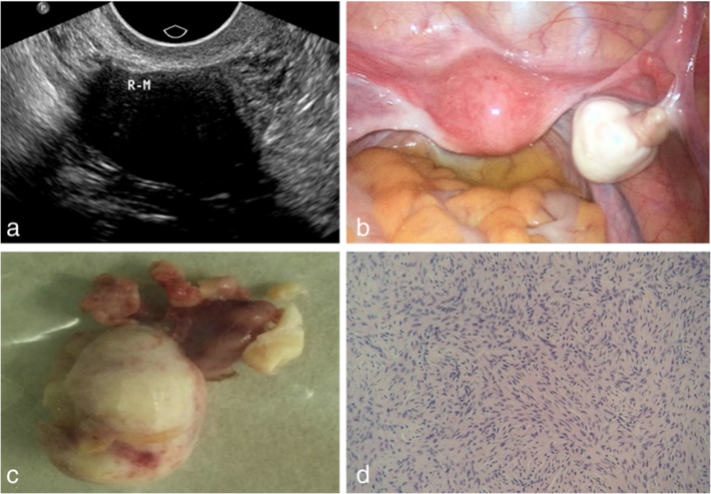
A 53-year-old woman with a pelvic mass discovered by routing physical examination. a On ultrasound examination, a 28 mm*22 mm*26 mm well-circumscribed hypoechoic mass was observed in the right ovary, with posterior echo attenuation. b On laparoscopy surgery, right ovarian was hard and enlarged, the surface smooth, having a good mobility; in the pelvic, a small number of pale yellow ascites were observed. c Pathologically, right ovarian was enlarged, with tough textures and grayish white surfaces. d Pathological findings confirmed thecofibroma in right ovarian
